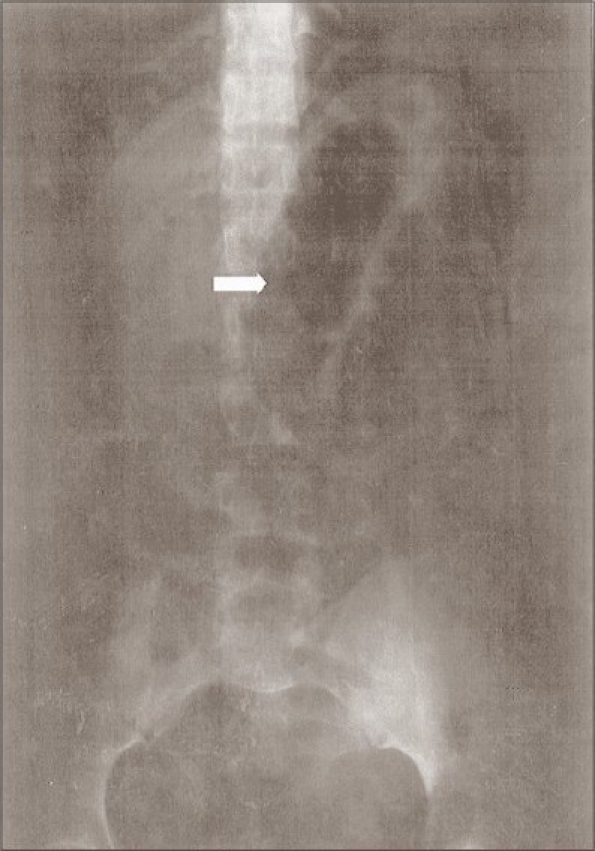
Plain X-ray abdomen showing dilated small bowel loops
